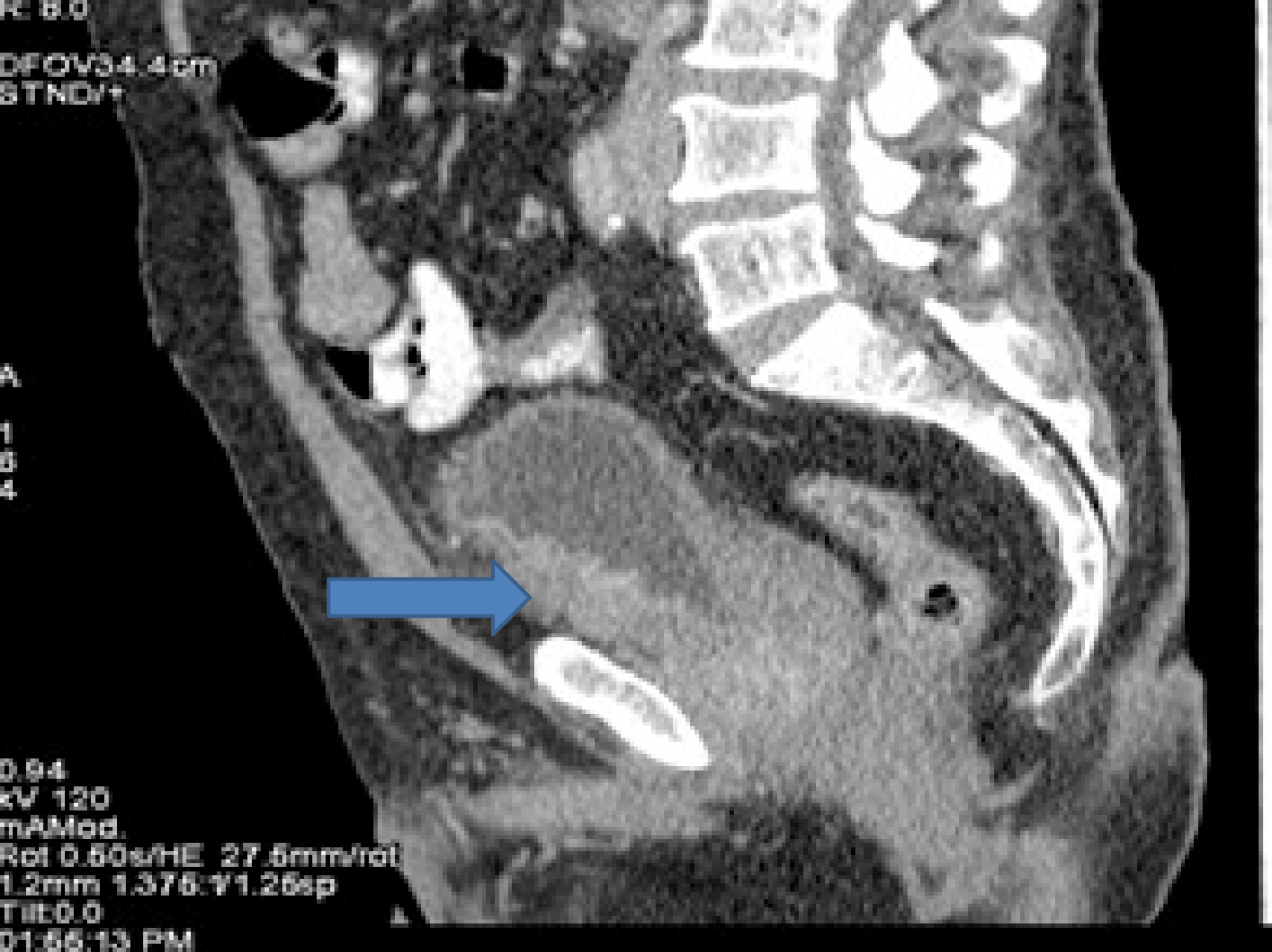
Computed tomography (CT) showing bladder cancer involving inferior half of urinary bladder with perivesical extension.
